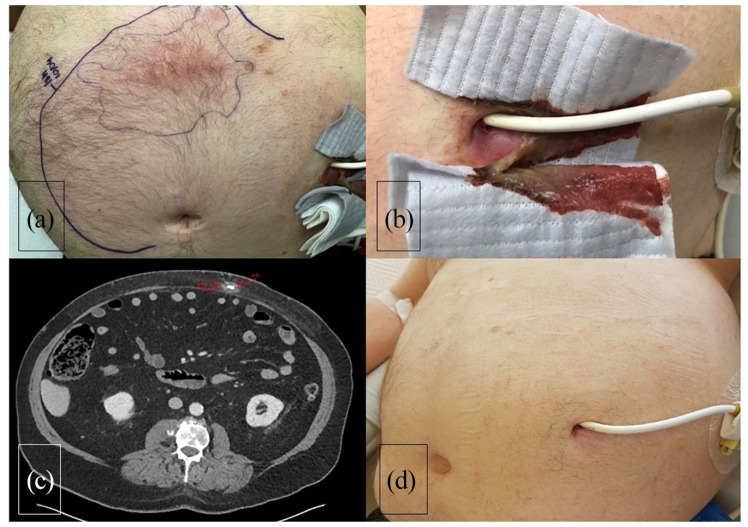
(a) Thoraco-abdominal CT shows infiltration and lobulated liquid collection
